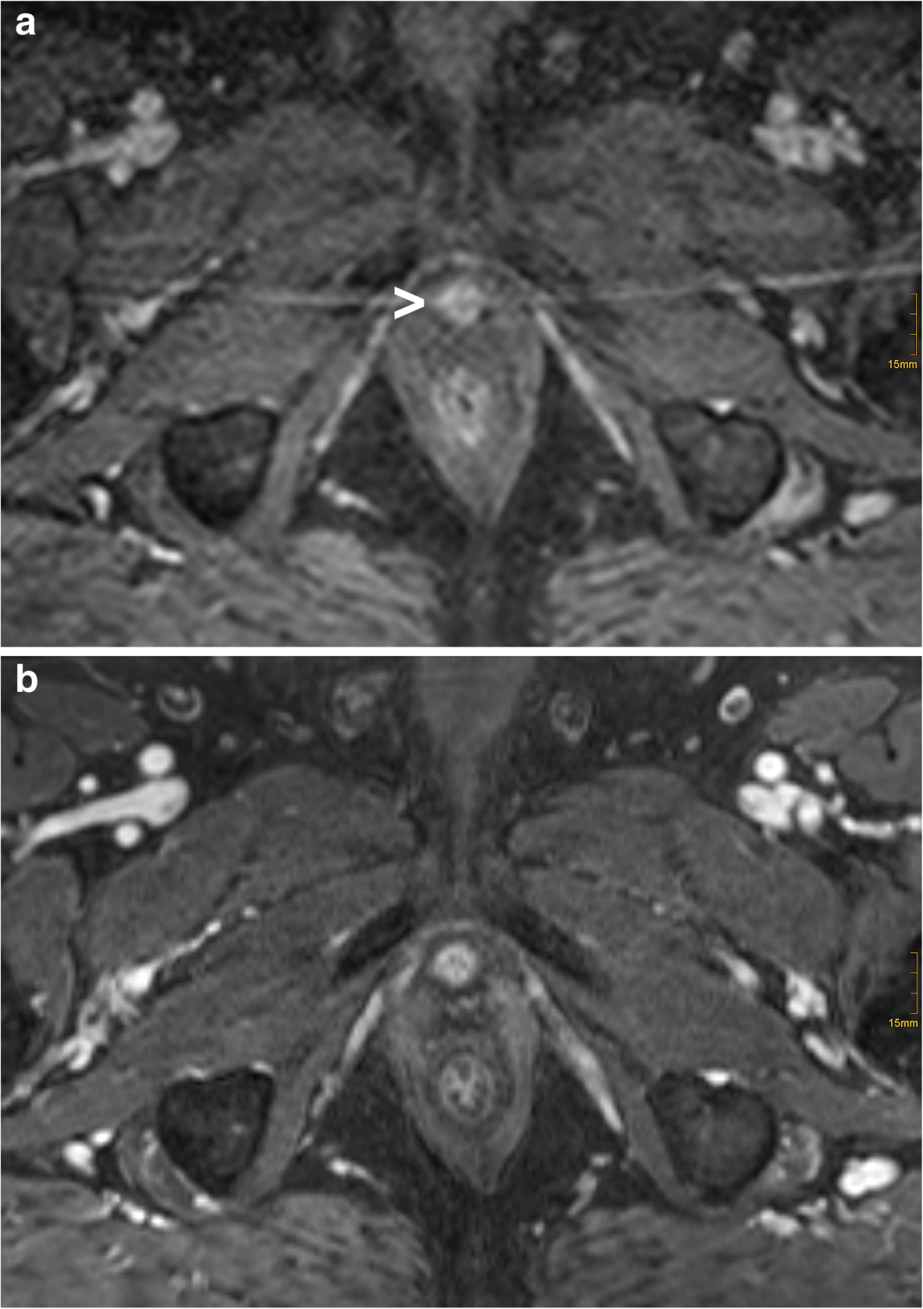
Contrast enhancement at the anterior aspect of the proximal urethra immediately below the vesicourethral anastomosis on pre-RT DCE-MRI which was not associated to the patient’s recurrent prostate cancer (a) (arrowhead). Post-RT DCE-MRI demonstrating no obvious change of this structure following RT (b).
